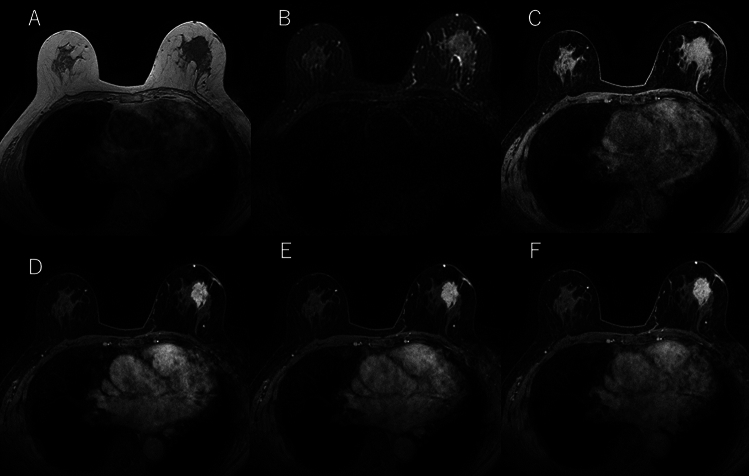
Representative MR images. A represents T1-weighted image, B represents fat-suppressed T2-weighted image, C represents fat-suppressed T1-weighted image, D represents early post-contrast phase, E represents late post-contrast phase, and F represents delayed post-contrast phase
